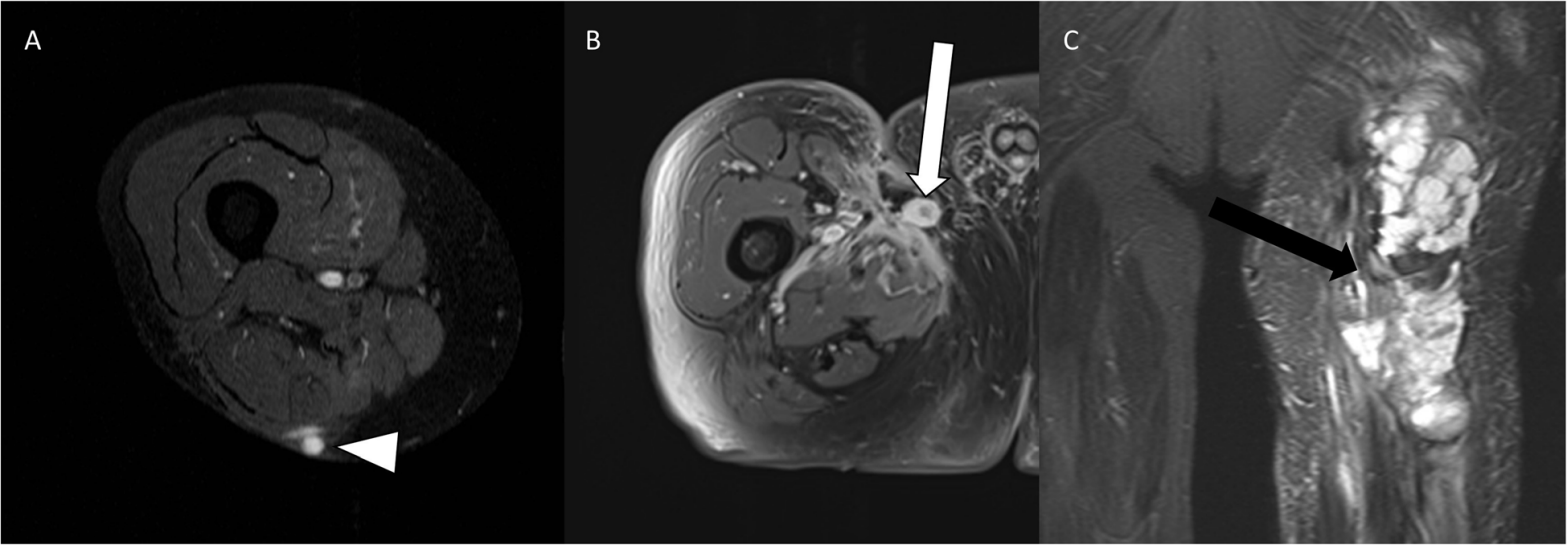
1.5-T MRI (a: contrast-enhanced T1-weighted image with fat suppression in axial view of the thigh; b: Contrast-enhanced T1-weighted image with fat suppression in axial view of the groin; c: Proton density weighted image with fat suppression in coronal view of the thigh). a shows a small nodular recurrence with slight edema (white arrowhead). b presents an ovoid/nodular recurrence (white arrow), which can easily be misinterpreted as a lymph node. c shows a multilobulated recurrence (black arrow) with adjacent edema
